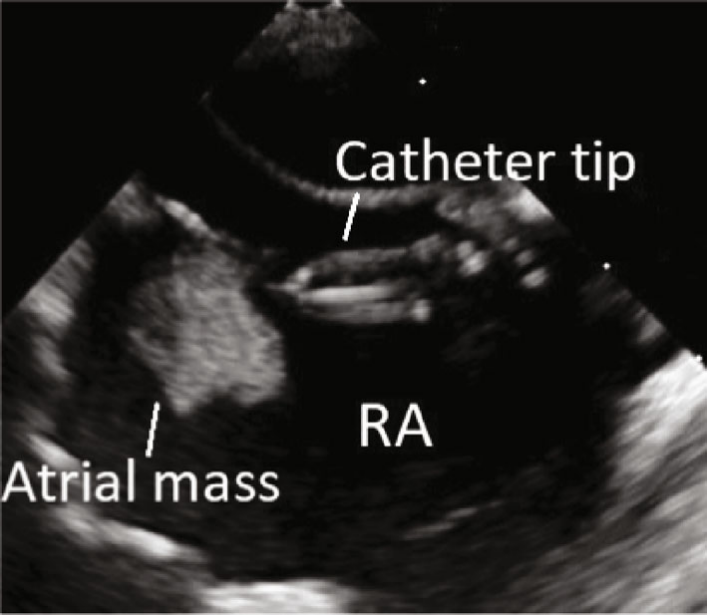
Transoesophageal echocardiography demonstrating vascular catheter tip abutting a right atrial mass.
